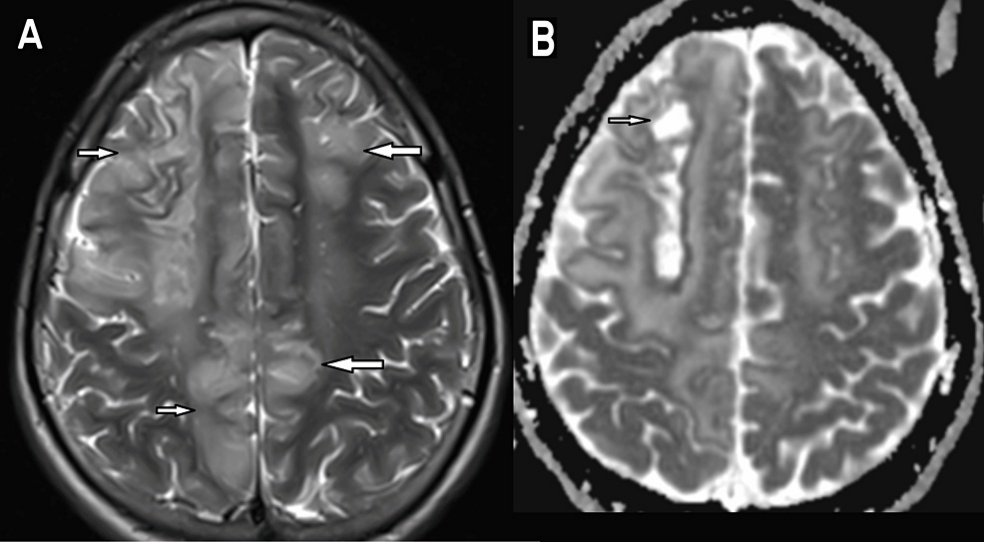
(A) T2-weighted imaging demonstrating post-inflammatory resolution with residual high signal; (B) Apparent Diffusion Coefficient (ADC) map normal diffusion facilitation
